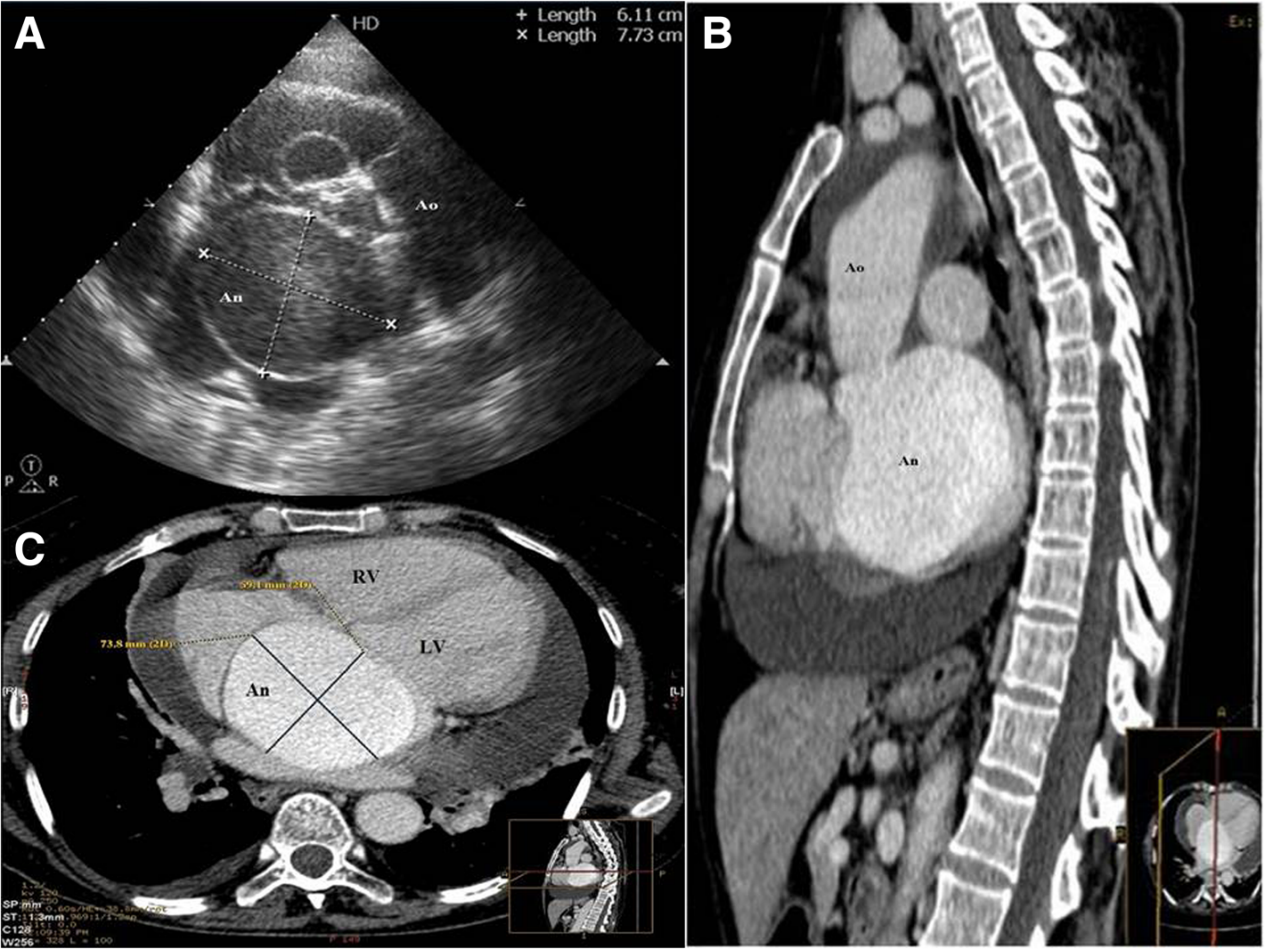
a. Transesophageal echocardiography demonstrating a giant aneurysm of the noncoronary sinus. b. Unruptured aneurysm of the noncoronary sinus. c. A giant aneurysm of the noncoronary sinus measuring 74×60 mm. *An* aneurysm of the coronary sinus, *Ao* aorta, *LV* left ventricle, *RV* right ventricle
